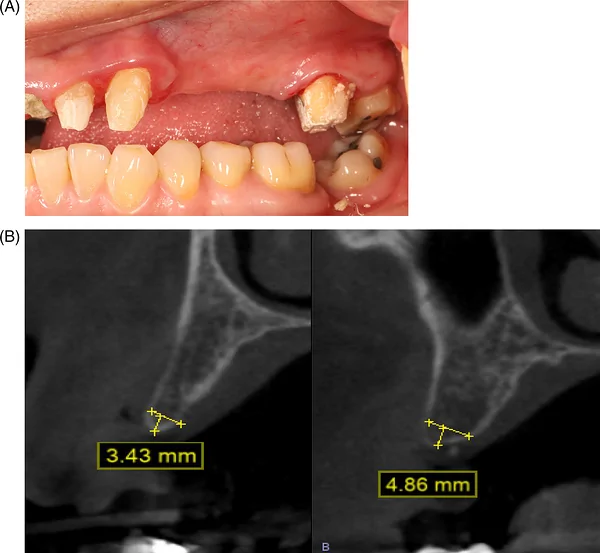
(A) Clinical presentation of the edentulous area planned for guided bone regeneration. (B) Preoperative CBCT image. Demonstrates a narrow alveolar ridge in width, around 3.43 mm on site #12 and 4.86 mm on site #14.
